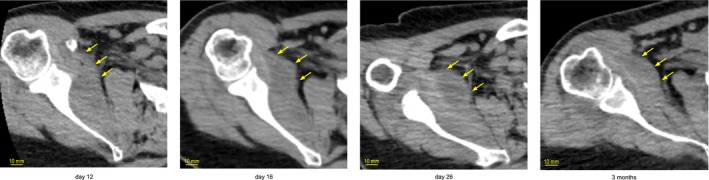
Plain computed tomography images of the abscess around the right shoulder joint. Axial computed tomography images (5‐mm slices) were taken at 12 days (admission), 16 days, 26 days, and 3 months. At the time of admission, the abscess measured 50.8 × 23.8 × 40.8 mm and appeared as a low‐density fluid collection with air inside. After 3 weeks of treatment, the abscess had decreased in size to 35.3 × 19.3 × 27.5 mm and had become organized.
